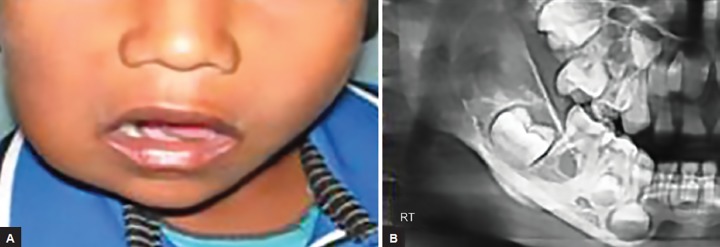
Preoperative photograph and orthopantomogram
